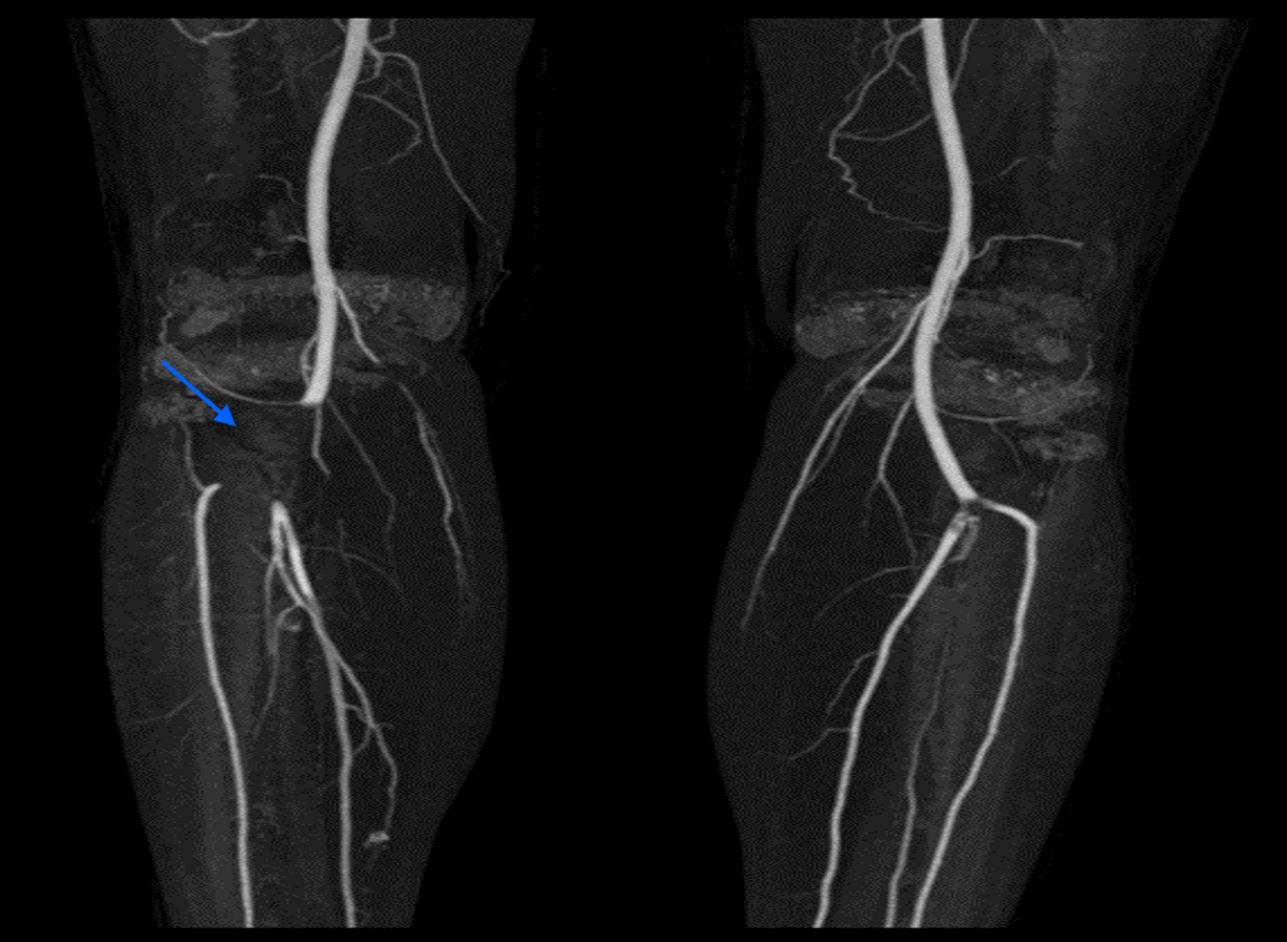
CT angiogram showing a partial right popliteal artery block.
